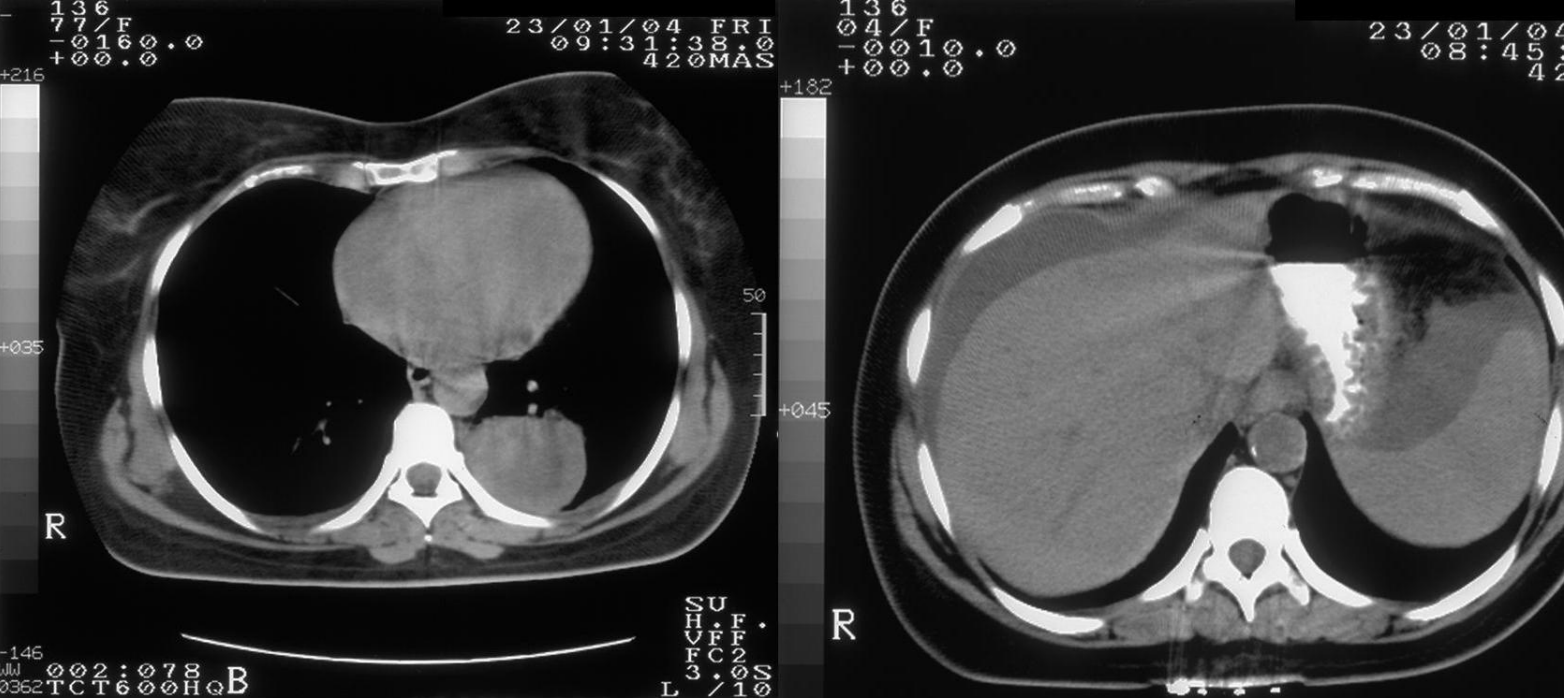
Thoracic and abdominal CT scans of the patient at initial presentation.
